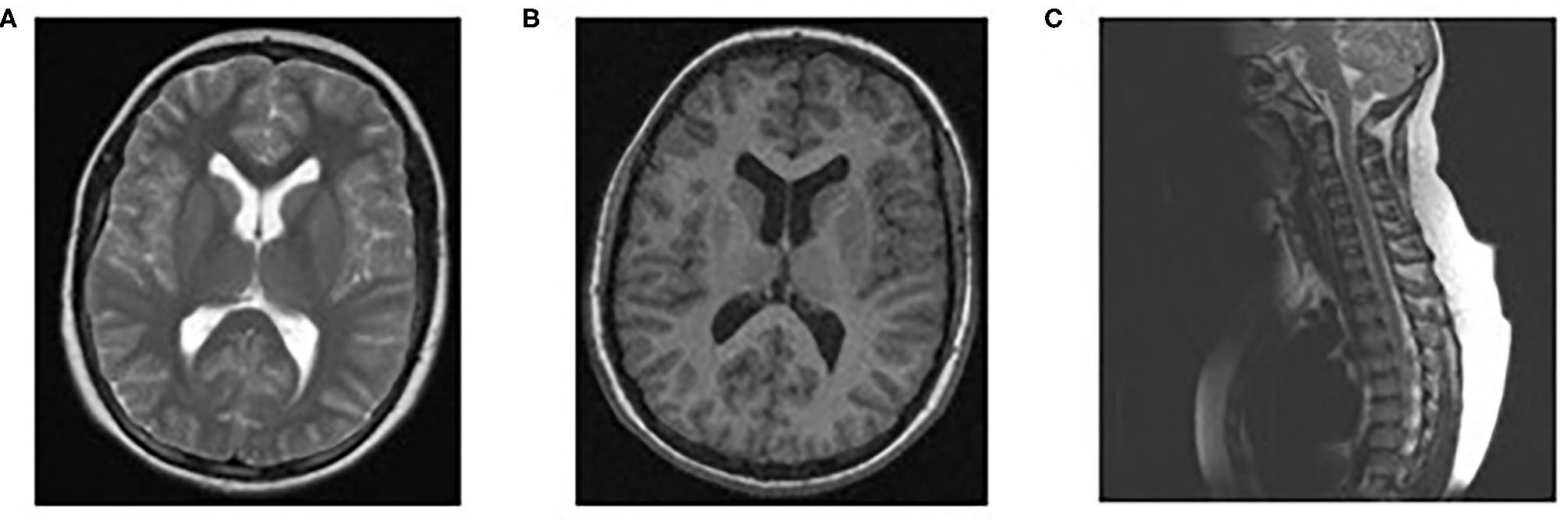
Normal findings of brain/spinal MRI. MRI brain scan T1 (B) and T2 (A), MRI spine sagittal (C).
